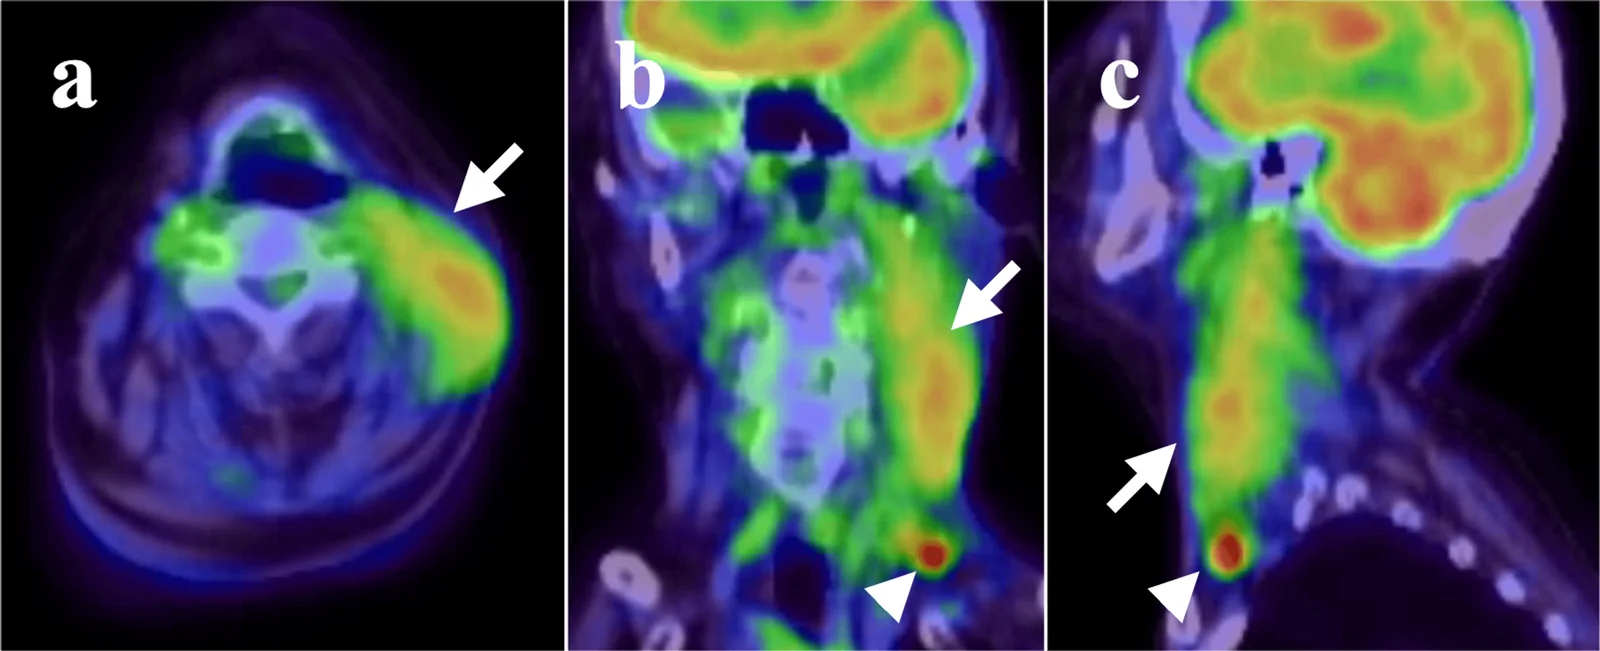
FDG-PET/CT images of the patient's neck obtained nine days after SBRT (a–c) The left cervical lymph node metastasis showing moderate FDG uptake (white arrow). (a) Axial, (b) coronal, and (c) sagittal views. (b, c) An unirradiated left supraclavicular lymph node metastasis showing intense FDG uptake (white arrowhead). FDG; 2-deoxy-2-[¹⁸F]fluoro-D-glucose, PET; positron emission tomography, CT; computed tomography, SBRT; stereotactic body radiotherapy.
